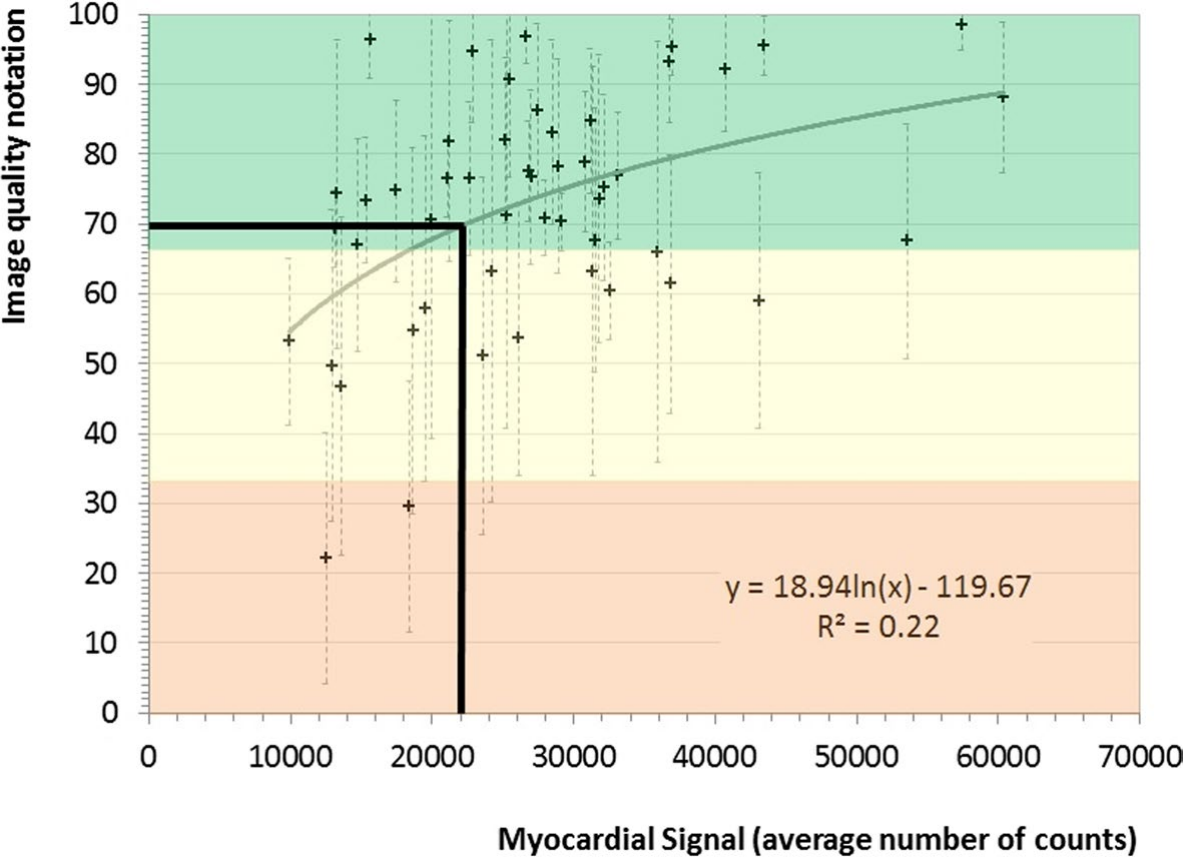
Image quality of the uncorrected stress-test SPECT images of the 50-patient subcohort. The image quality at different myocardial signals was scored by seven nuclear physicians. Black crosses and gray error bars, respectively, indicate the average and standard deviation of the image quality scores recorded by the physicians. The orange, yellow, and green areas indicate poor (score < 33), intermediate (score 33–66), and good (score > 66) image quality, respectively. The gray curving line represents the logarithmic regression of the data. The black landmarks show how the chosen MS threshold of 22,000 counts corresponds to the expected image quality. An image quality score of around > 70 signifies good image quality
